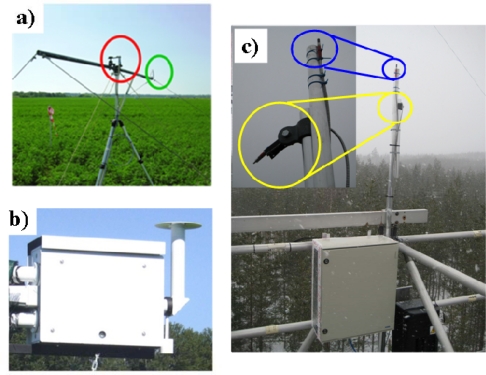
Pictures of the systems installed in the field. (a) Optics of the MRI mounted on a tripod (cosine receptor head in red; bare fiber position in green). (b) Picture of the HSI box installed in field. (c) Two Dual Field of View (DFOV) system installed at Hyytiälä site, Finland (upward pointing cosine receptor in blue; downward fiber in yellow).
